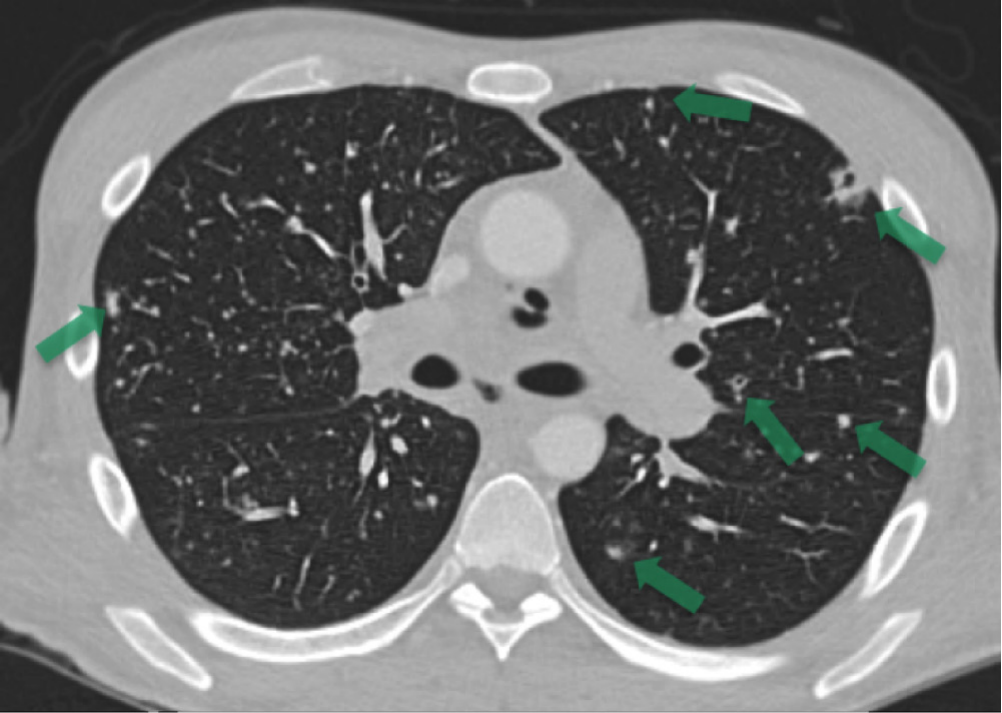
Chest CT on admission revealed multiple cavitary and non-cavitary lung nodules (arrows), suspicious for mycobacterial infection.
